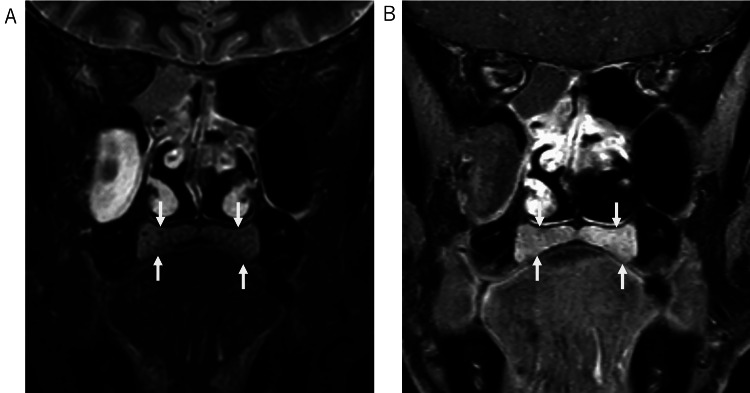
Thickening of the hard palate mucosa. Fat-saturated T2-weighted image (A) and post-contrast fat-saturated T1-weighted image (B) demonstrate diffuse thickening of the hard palate mucosa.
